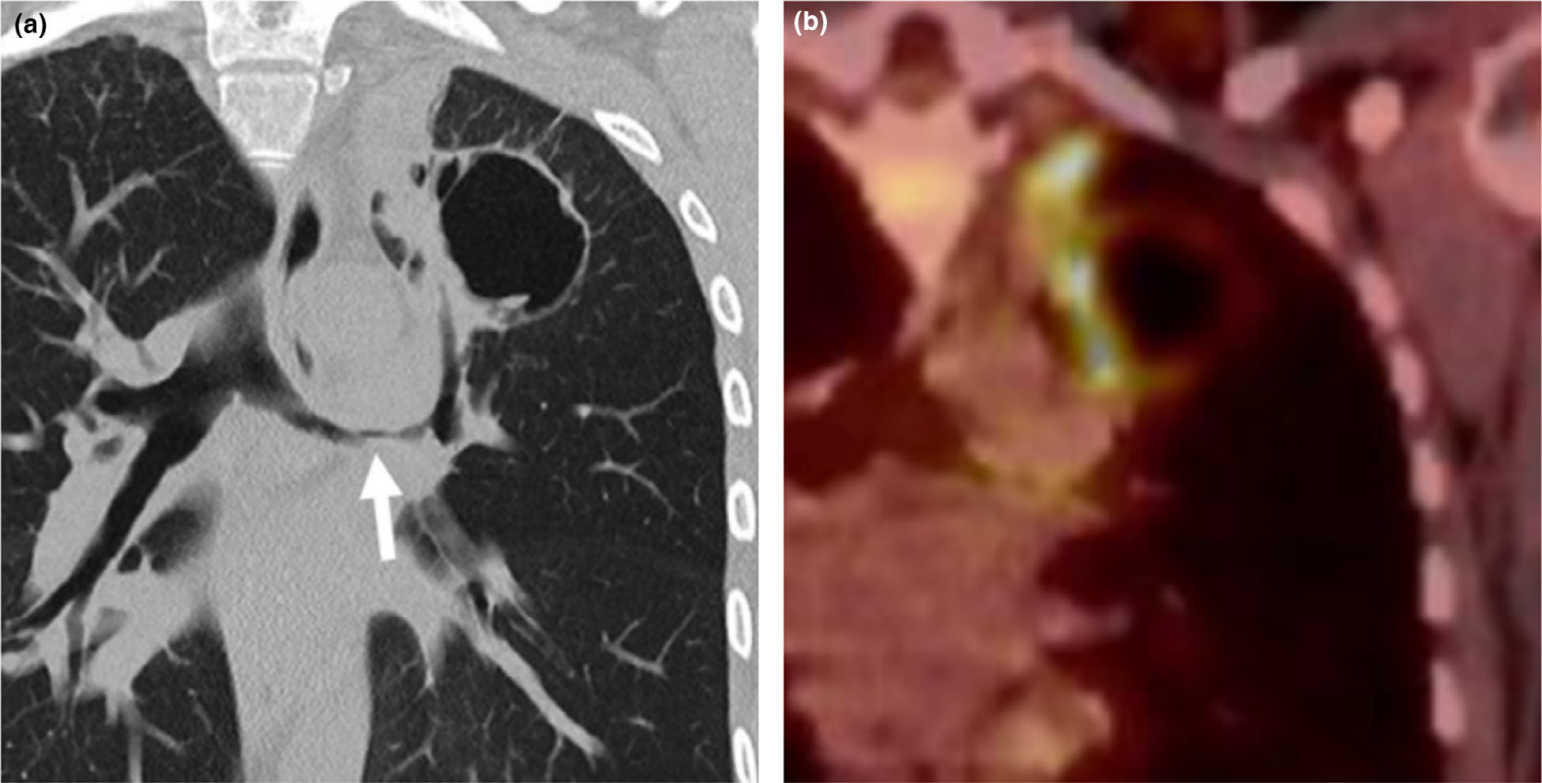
Coronal (a) reformats of a non‐contrast CT of a 34‐year‐old female demonstrating a lobulated cavitating nodule in the left upper lobe with irregular nodular wall thickening as well as marked irregular narrowing of the left main bronchus (white arrow). 18F‐Fluorodeoxyglucose (FDG) PET with a corresponding coronal (b) image showed avidity in its wall and septum, more marked along its medially thickened wall indicating this lesion was metabolically active. Subsequent resection of this lesion confirmed this was a GPA lesion.
